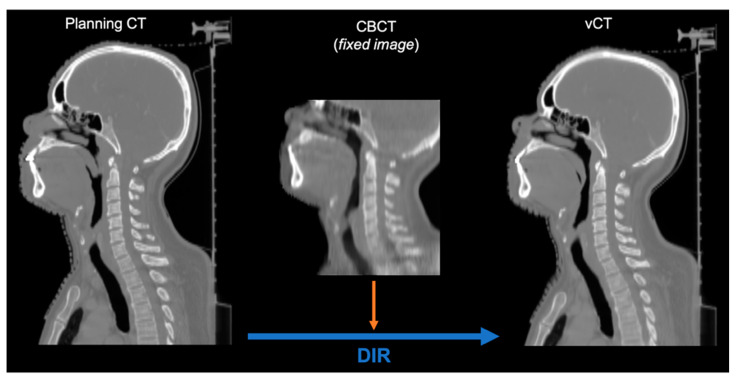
Preparation of a vCT based on a fraction-specific CBCT.
